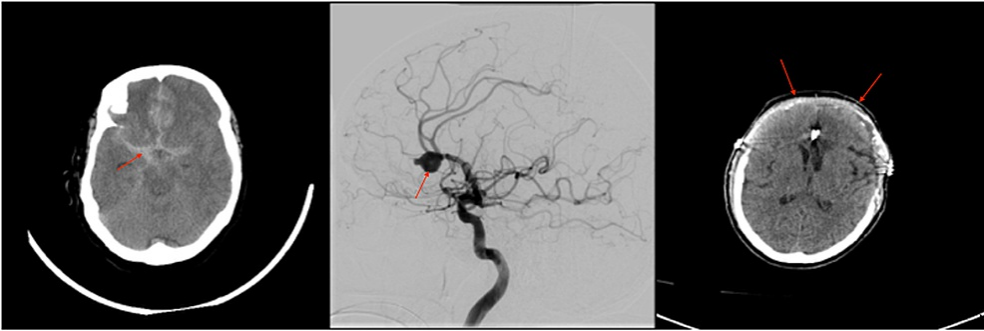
Imaging related to the clinical course of patient 1. (A) Head CT obtained on presentation demonstrated SAH secondary to a ruptured right pericallosal aneurysm. (B) Angiogram obtained after presentation demonstrating a large right pericallosal aneurysm. (C) Post-operative head CT after bilateral DCHC for refractory elevated ICP. SAH, subarachnoid hemorrhage; DCHC, decompressive hemicraniectomy; ICP, intracranial pressure
